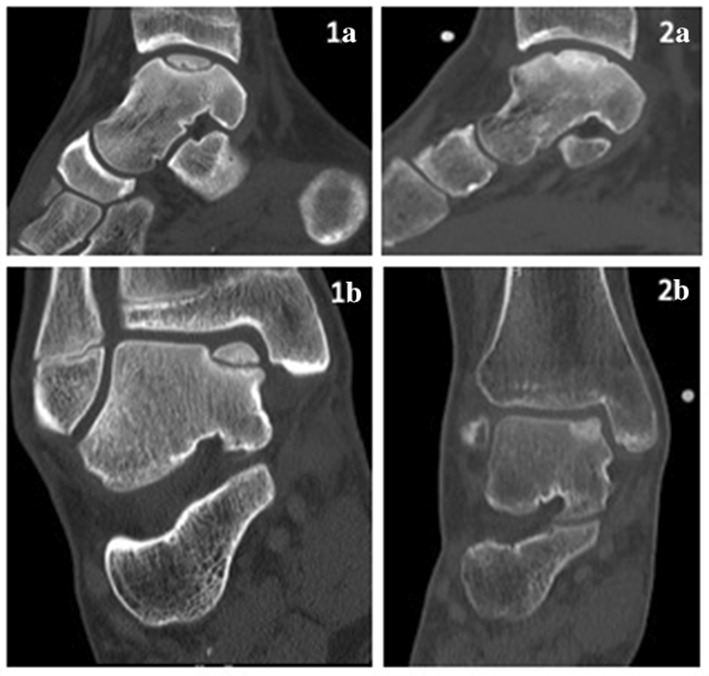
Example of a pre-operative sagittal (1A) and coronal (1B) CT scan of the OCD of a 15-year-old patient and sagittal (2A) and coronal (2B) CT scan from the same patient 1 year after the LDFF procedure
